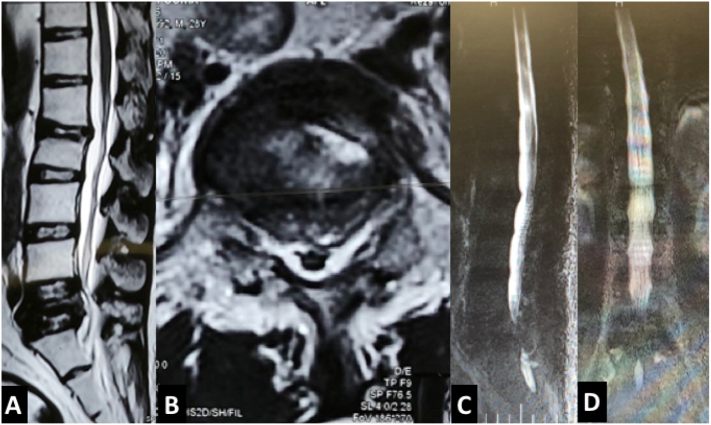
MRI scanning revealed double line sign in the place of 5th vertebral body.
